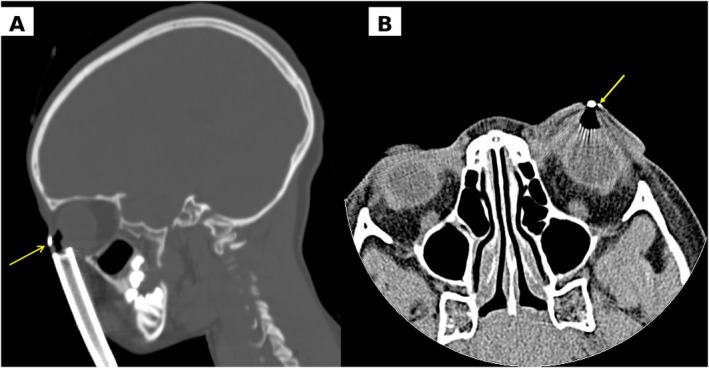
Non‐contrast orbital computed tomography images: (A) sagittal reformatted bone‐window image; (B) axial soft‐tissue‐window image. Yellow arrows indicate the metallic foreign body embedded within the left upper eyelid and confined to the periocular soft tissues. The globe is intact, with no orbital wall fracture or retrobulbar hemorrhage.
